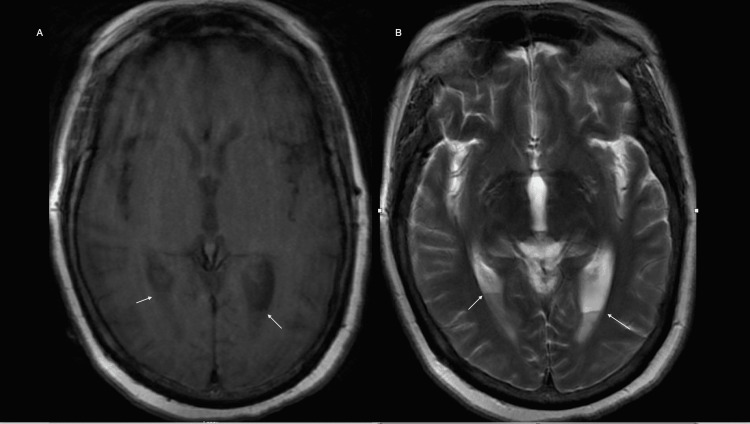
MRI images. (A) Axial T1 image showing the layering of pyogenic material in bilateral occipital horns. (B) Axial T2 image showing the layering of pyogenic material in bilateral occipital horns. MRI: magnetic resonance imaging
